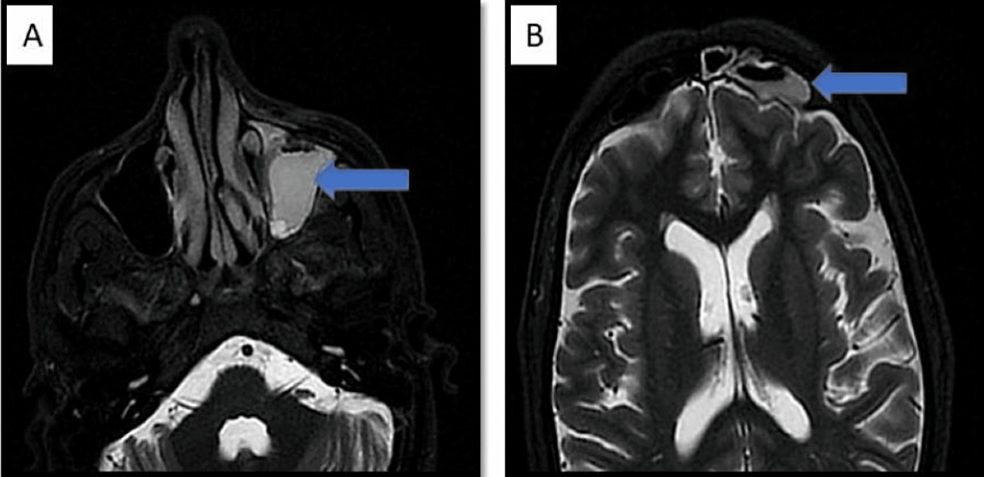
Sinonasal involvement Image A and B: T2 stir axial MR image shows mucosal thickening noted involving left maxillary, ethmoidal, and frontal sinuses, with these sinuses showing air fluid level.
